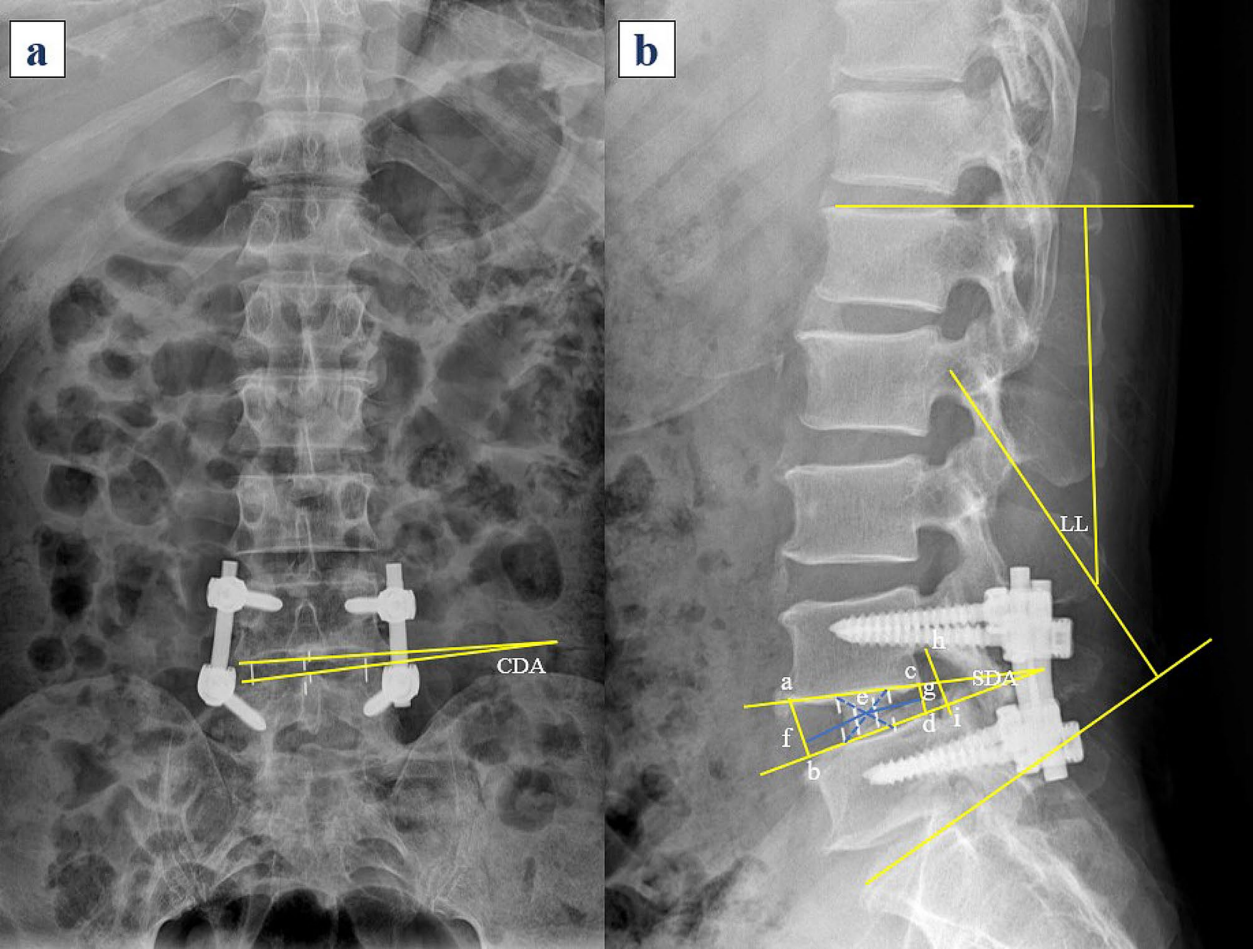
Measurement of radiological data. (a) The coronal disc angle (CDA) was defined as the angle between the lower endplate of the superior vertebra and the upper endplate of the inferior vertebra on anteroposterior X-ray images. (b) The sagittal disc angle (SDA) was defined as the angle between the lower endplate of the superior vertebra and the upper endplate of the inferior vertebra on lateral X-ray images. Line segment ab represents the anterior height (AH) of the intervertebral space, while line segment cd represents the posterior height (PH) of the intervertebral space. The disc height (DH) is defined as the average of the AH and PH. The foraminal height (FH) is defined as the distance between the highest point (h) of the superior pedicle and the lowest point (i) of the inferior pedicle. LL is defined as the angle formed by the perpendicular lines from the superior endplate of the first sacral vertebra (S1) and the superior endplate of the first lumbar vertebra (L1). Point e represents the center of the cage, and the location of the cage is defined as the ratio of the distance from the center point e to the anterior edge of the disc space (ef) to the distance from the center point e to the posterior edge of the disc space (eg). CDA: coronal disc angle; AH: anterior height; PH: posterior height; DH: disc height; SDA: sagittal disc angle; FH: foraminal height; LL: lumbar lordosis; SD: standard deviation; IQR: interquartile range
